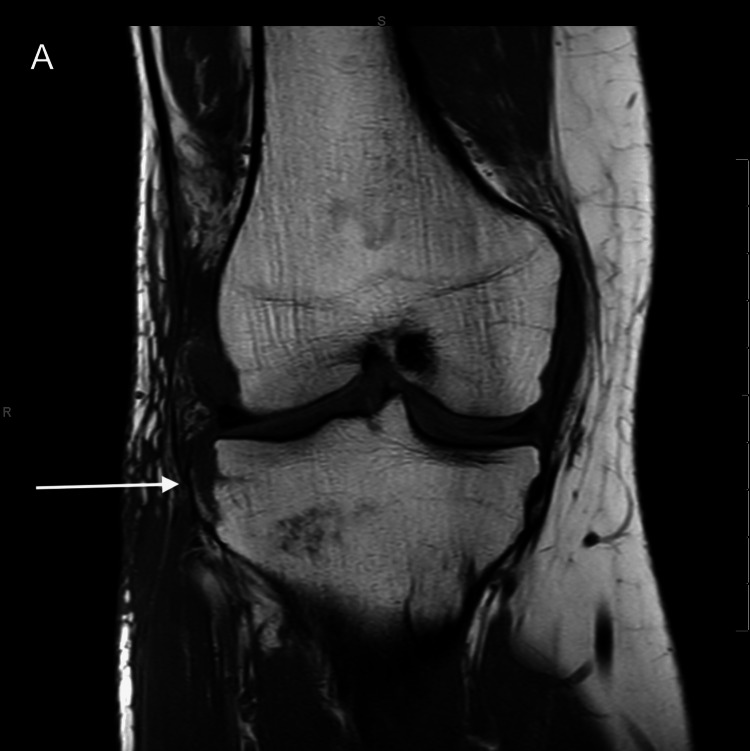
(A) Coronal T1-weighted image shows the Segond fracture (arrow).
